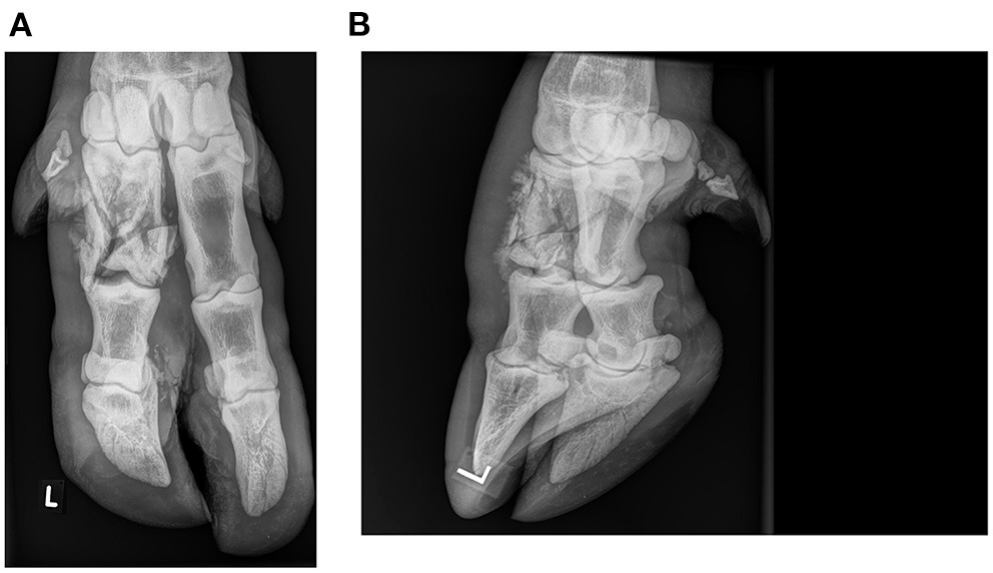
Plantarodorsal (A) and oblique lateral (B) radiographs of a multifragmentary fracture of the lateral proximal phalanx in an adult female European bison (*Bison bonasus*): One month after the injury and immediately before applying an orthopedic claw block.
